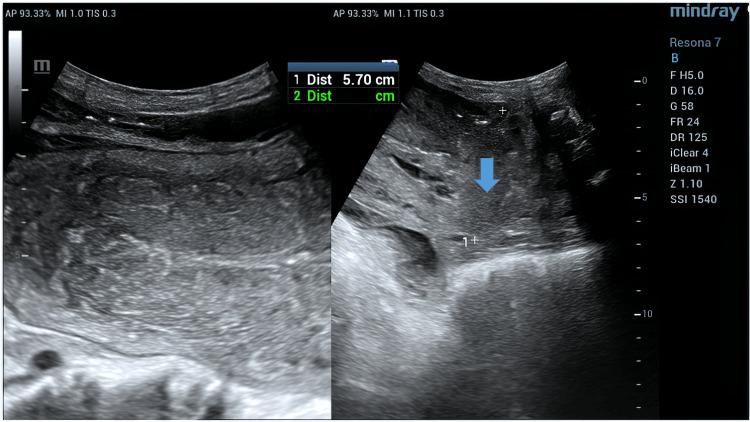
Sagittal pelvic ultrasound slices. An isthmocorporal uterine parietal discontinuity (arrow) communicating the uterine cavity with the pelvis.
